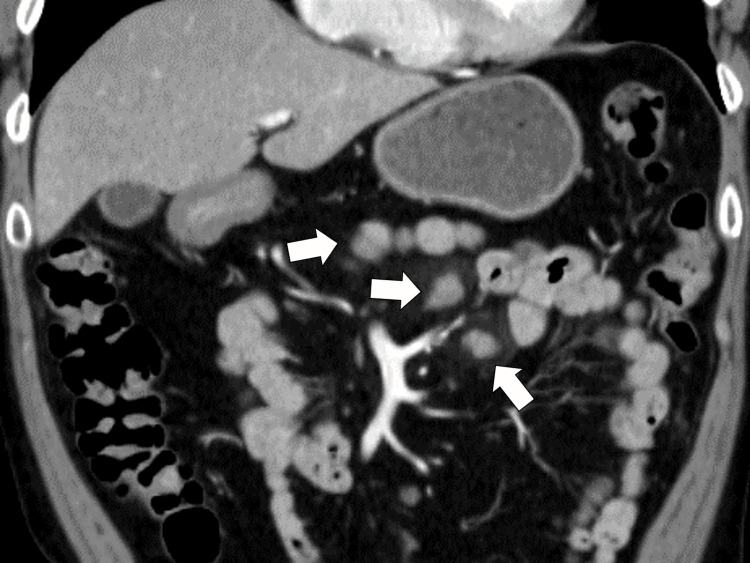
Abdominal computed tomography with contrast clarifying local lymphadenopathy around the stomach without any extravasation on the stomach's mucus (white arrows).
